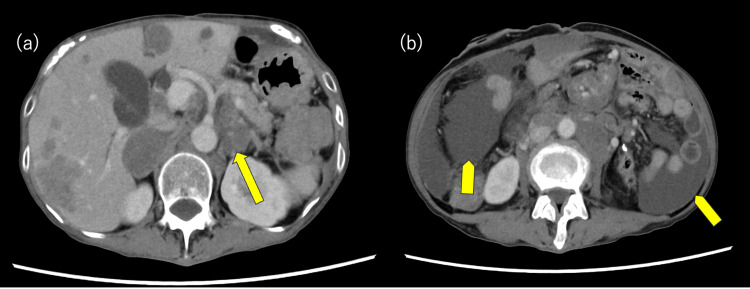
Abdominal CT scan performed one month after ABBV-400 administration. (a) An increase in lymph node enlargement (arrow); (b) the appearance of ascites (arrowhead).
